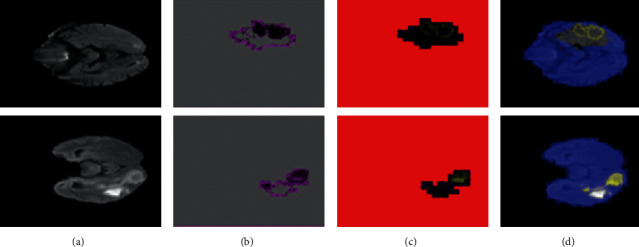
Proposed method segmentation outcomes on Challenge BRATS-2020 (a). Original MRI slices (b). Three-dimensional segmentation (c). The segmented region is mapped on input slices (d).
